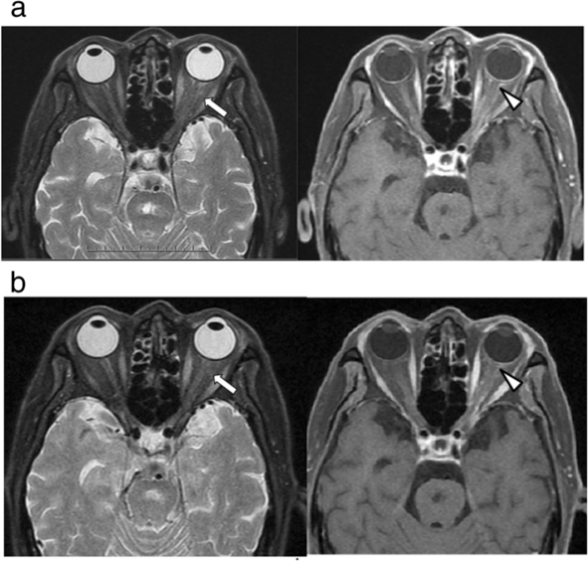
Orbital MRI findings. a On day 6 of admission, there was enhancement and swelling of the left extraocular muscles (arrows) on T2-weighted images; and mild enhancement of the left optic nerve sheath (arrowhead), left posterior orbital wall, and left orbital apex on contrast-enhanced T1-weighted images. b At 180 days after the onset of symptoms, there were no significant changes compared with the MRI findings on day 6 of admission.
